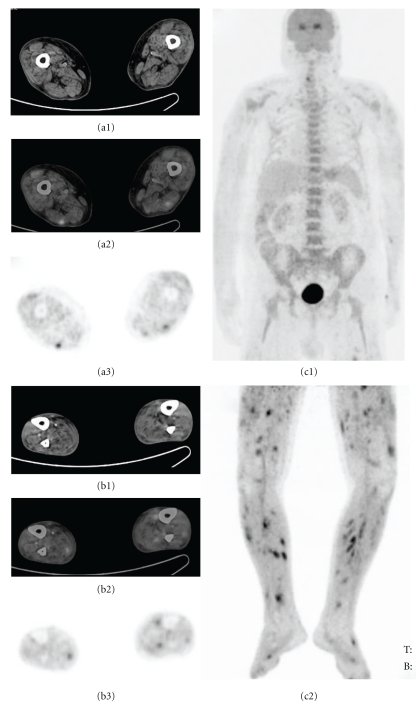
PET-CT of a 77-year-old man with FUO. Transaxial slices of CT (a1, b1), fusion (a2, b2), and PET (a3, b3), and anterior whole body (c1), lower extremities (c2) MIP images revealed multiple foci in the walls of vessels. Nonspecific vasculitis was diagnosed by biopsy.
